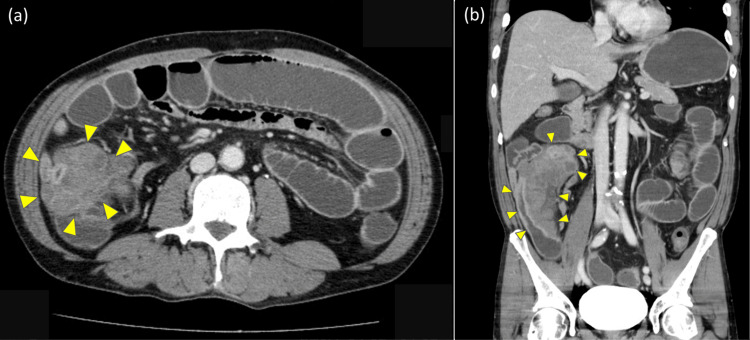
Abdominal contrast-enhanced CT findings. (a) Axial image showing dilatation of the proximal small intestine and extraluminal mass lesions adjacent to the right small intestine causing luminal stenosis. (b) Coronal image demonstrating extrinsic compression of the right small intestine by the extraluminal mass lesion. CT: computed tomography.
